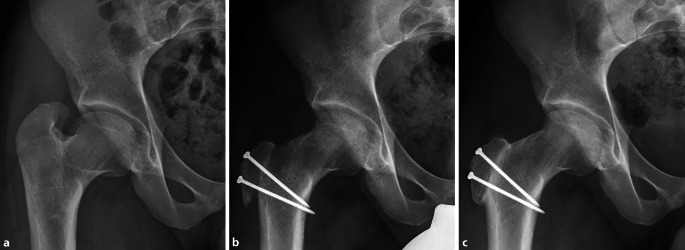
a Example of a 30-year-old man with typical femoral head deformity following LCPD (Legg-Calve-Perthes Disease) with an enlarged, aspherical head and a shortened neck. This results in a relatively high riding greater and lesser trochanter resulting in restricted range of motion and extraarticular impingement. b Following relative femoral neck lengthening, the greater trochanter was distalized, the base of it reduced to elongate the neck, the cam deformity was resected and in addition the lesser trochanter was distalized. Radiograph was performed at the 8‑week follow-up. c Radiograph at 1‑year following surgery with healed osteotomies, no signs of osteoarthritis or avascular necrosis
